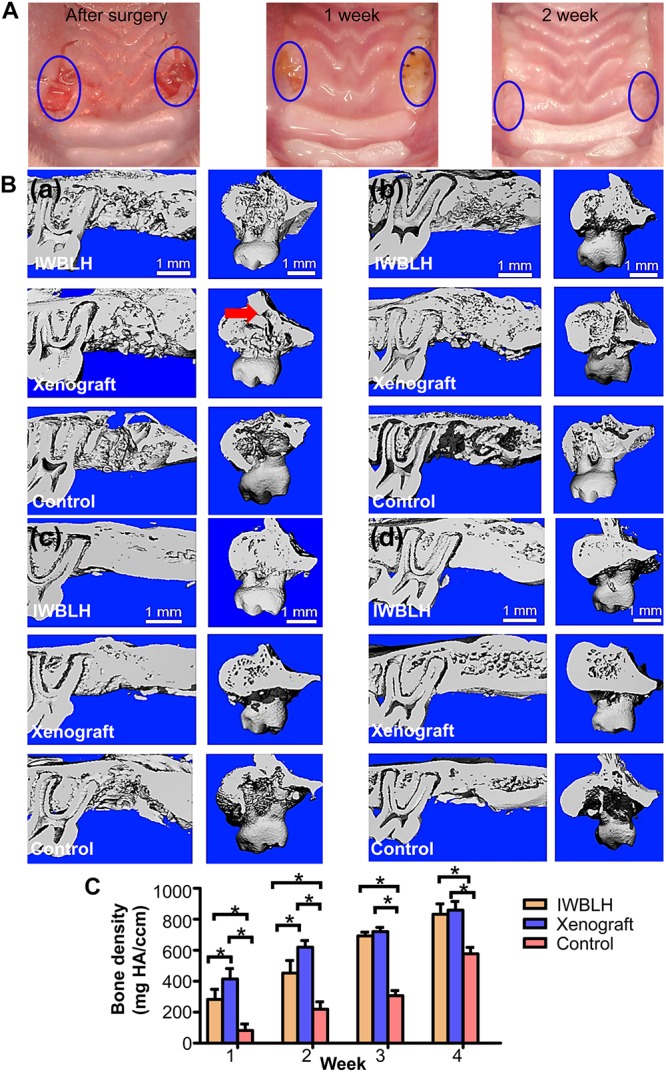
(A) The gross observation of tooth socket healing the after surgery. The left tooth socket was grafted with IWBLH, no bone substitute was grafted in the right socket. (B) The micro CT images of tooth socket after first week (a), second week (b), third week (c), and fourth week (d). The red arrow indicated the cavity that was not filled by the xenograft. (C) The bone density of tooth socket (*n* = 6. The bars show the mean ± SD, p* < 0.05).
